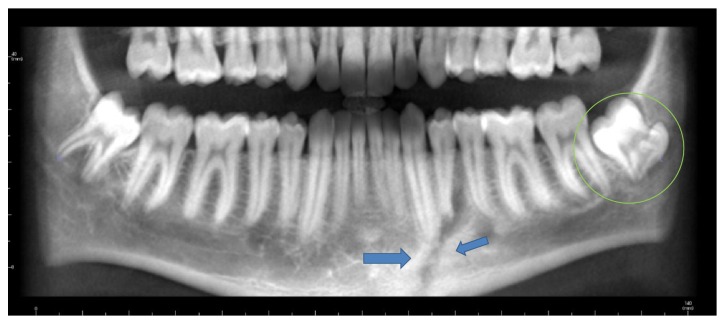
Panoramic radiograph showing concrescence in the lower left third molar region (green circle); additionally, fracture line (blue arrows) is noted in the premolar region (left side).
